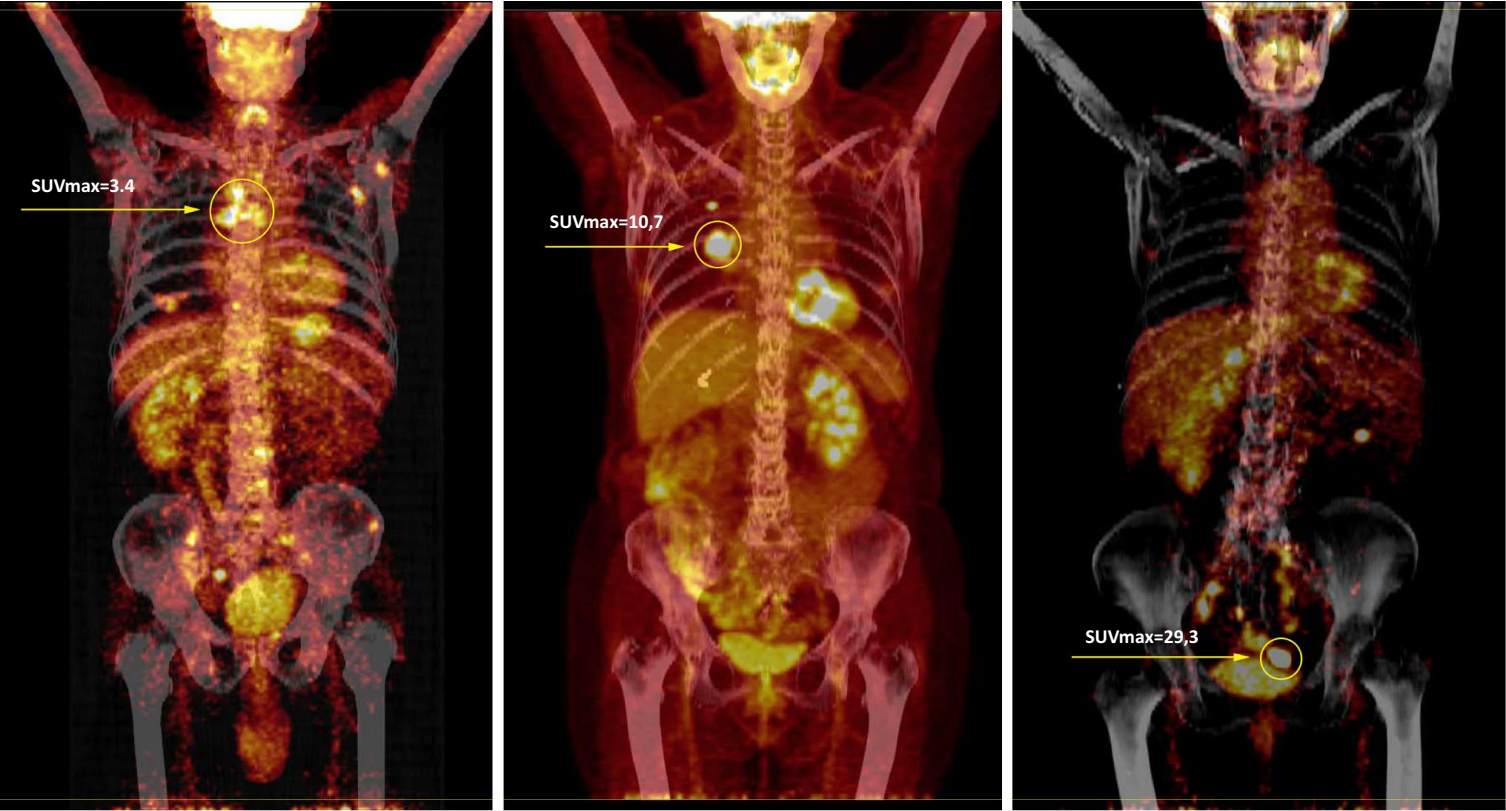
Sample FDG PET/CT images as a function of identified SUVmax cutoff points
